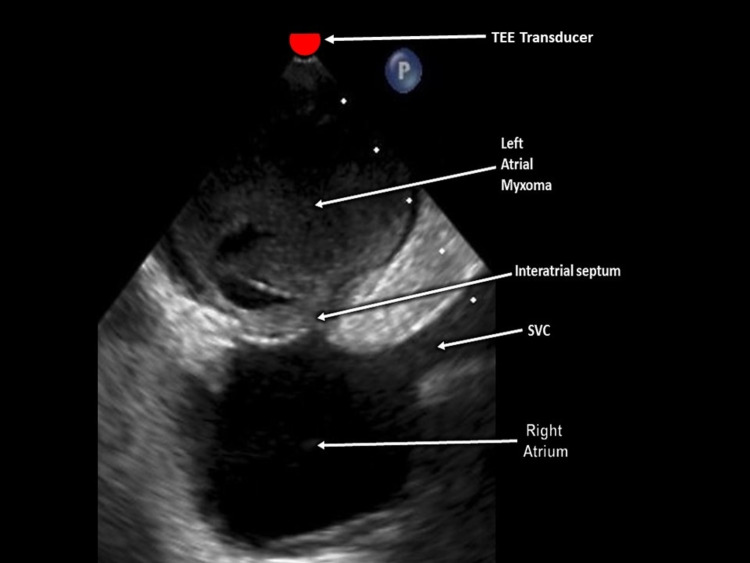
Transesophageal echocardiogram (TEE), left atrial myxoma SVC - superior vena cava
